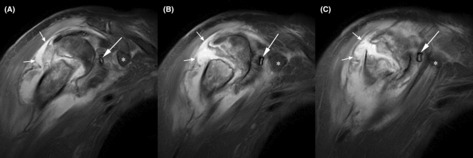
T2 MR images in axial view (A is most posterior, B is just anterior to A, and C is most anterior). Asterisks (*) indicate the coracoid process and the long arrows indicate the Jackson–Pratt drain and the short arrows indicate the abscess. The scan was obtained 13 days after the fracture.
